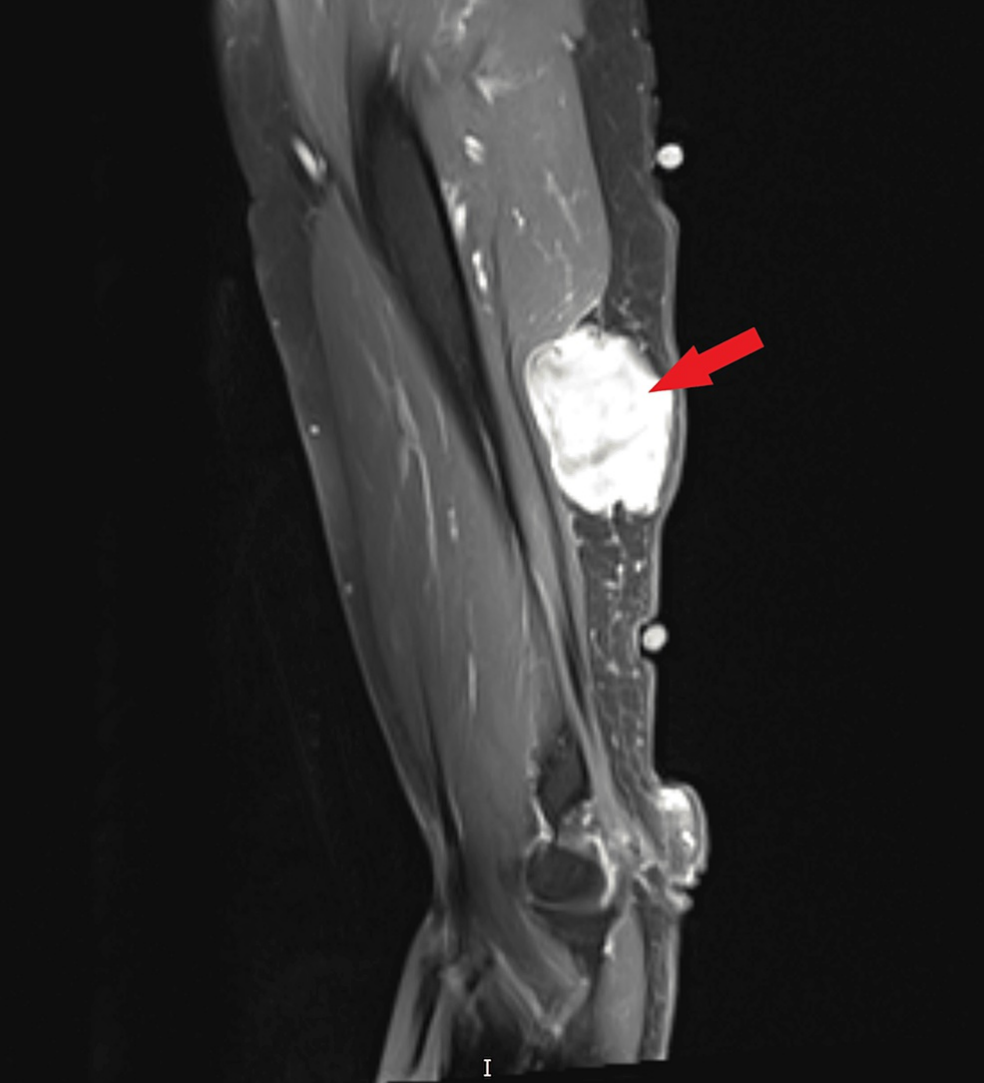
MRI of the humerus showing 5.2 cm soft tissue mass (red arrow) within the subcutaneous fat overlying the triceps region concerning for malignancy.
